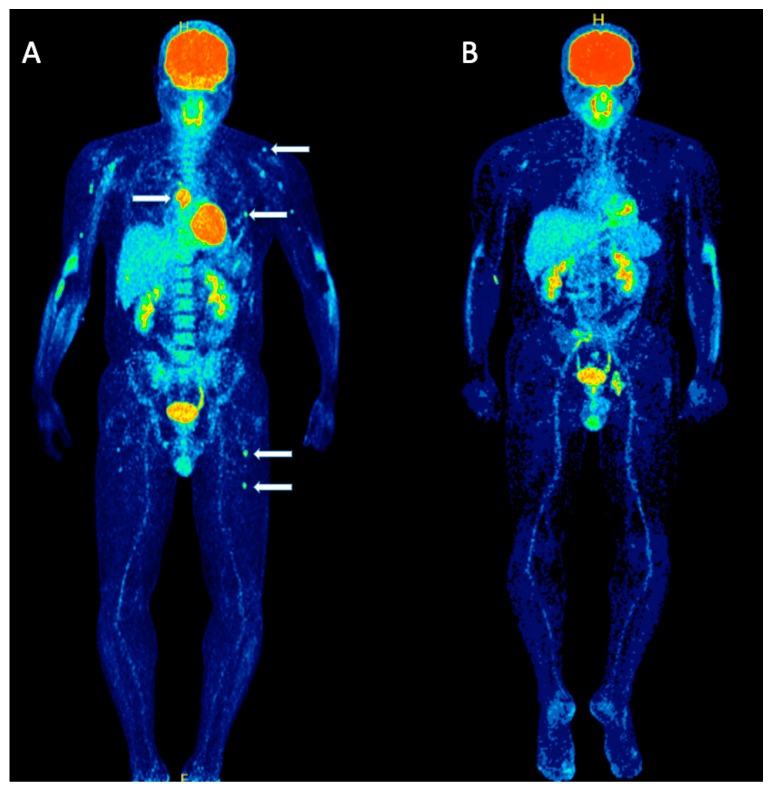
A 39-years old symptomatic MM patient scheduled for HDT and ASCT, undergoing ^18^F-FDG PET/CT before and after therapy. Maximum intensity projection (MIP) ^18^F-FDG PET/CT before therapy (A) revealed a mixed pattern of ^18^F-FDG uptake with intense, diffuse uptake in the axial skeleton and multiple, focal bone marrow lesions for example in the sternum, ribs, humerus, scapula and femur (arrows). Follow-up ^18^F-FDG PET/CT MIP after high-dose chemotherapy and ASCT (B) demonstrated a complete remission of both diffuse bone marrow uptake as well as focal MM lesions.
